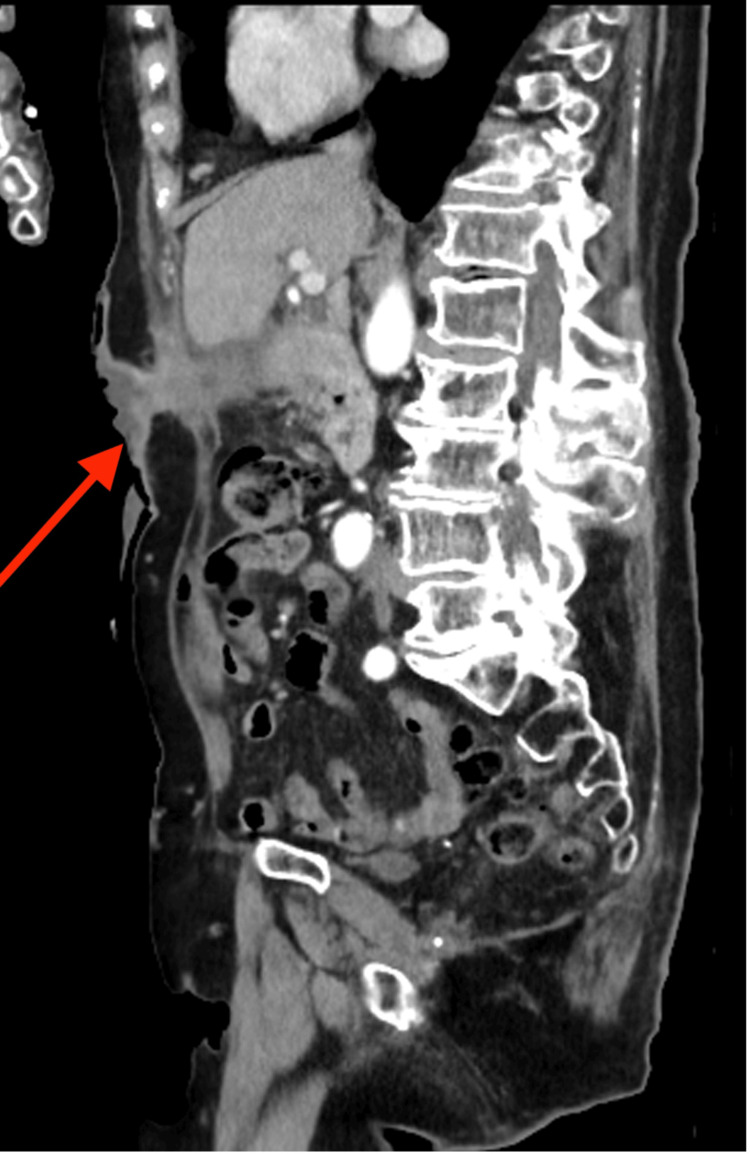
CT abdomen and pelvis with IV contrast (sagittal) showing a CCF along the drain tract (red arrow) CCF: cholecystocutaneous fistula; CT: computed tomography; IV: intravenous
